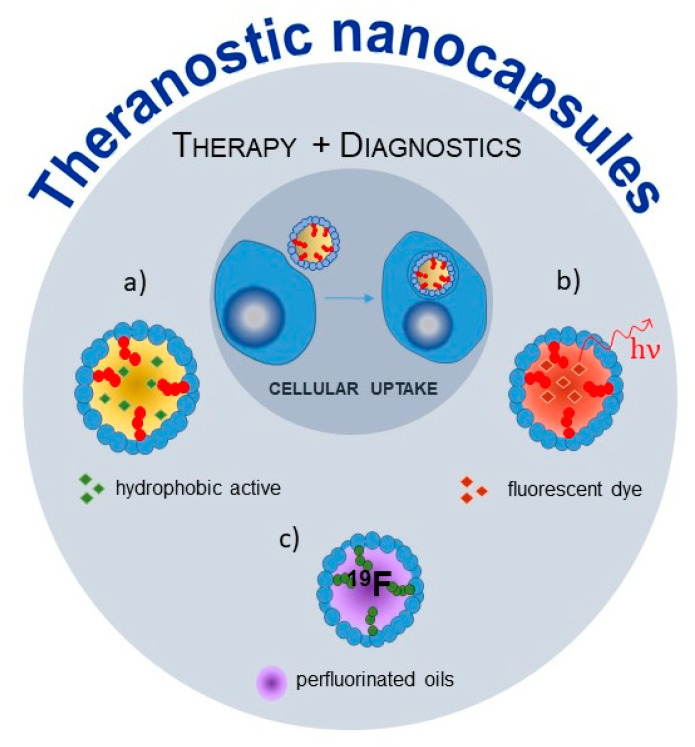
Functionalized nanocapsules for theranostic applications. The hydrophobically modified, polysaccharide-based nanocapsule encapsulating hydrophobic actives in oil core: (a) intracellular delivery of hydrophobic actives, (b) fluorescent dyes for imaging and capsules detection and (c) perfluorinated compounds for ^19^F NMR/MRI.
